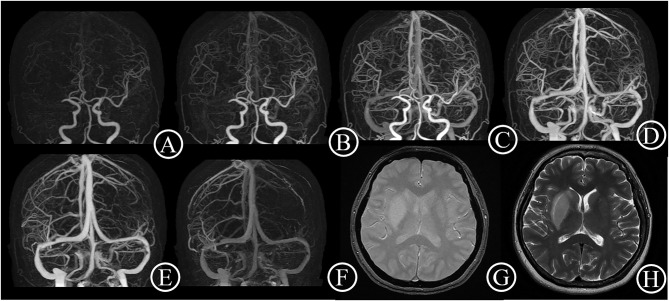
Good collateral case. One 48-year-old female patient suddenly suffered from inability to lift left upper limb for 30 min. She had an initial NIHSS score of 11 and a mRS score of 0 after 3 months. 4D CTA (A–F) showed occlusion of right middle cerebral artery, and complete collateral circulation before venous phase. Thus, the patient got a 4D CTA collateral circulation score of 4. The T2 star (G) and T2WI (H) on day 6 showed a small acute infarct lesion without hemorrhage transformation.
